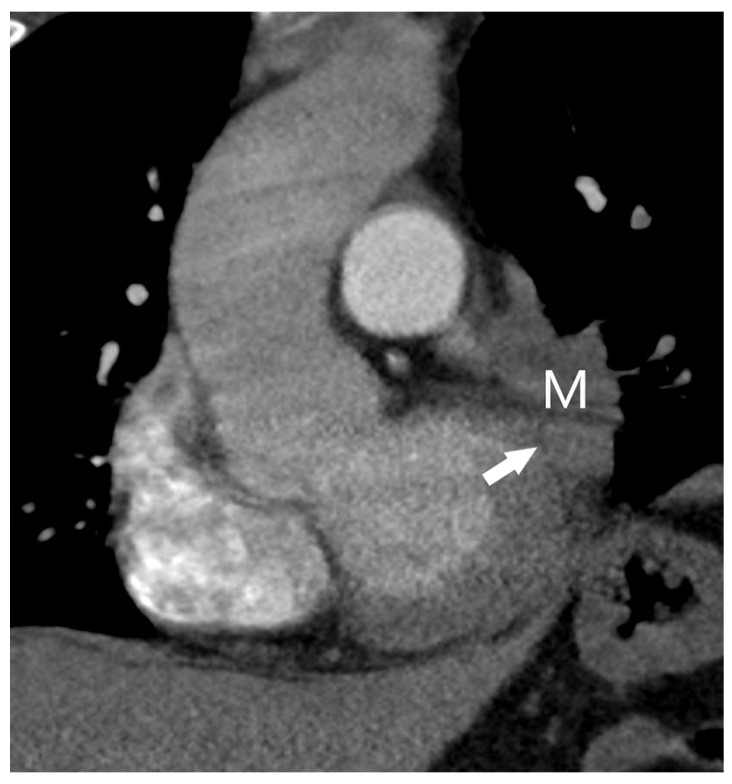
The T3 category: Coronal reformatted CT image (soft-tissue window) showing a mass (M) invading the pericardium (arrow). The left hemidiaphragm is elevated, indicating invasion of the left phrenic nerve.
